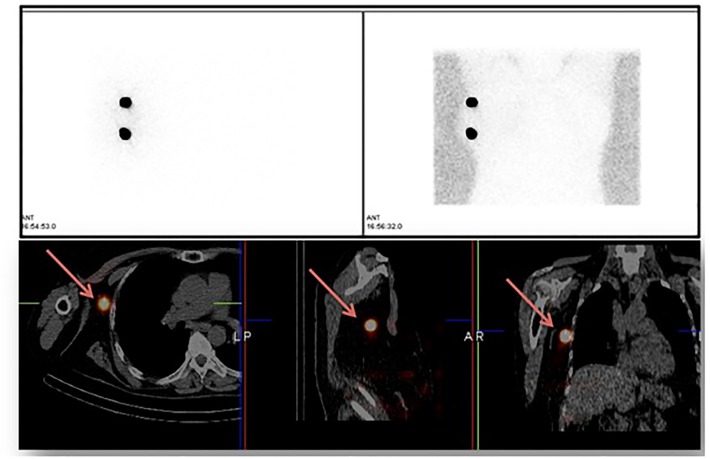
PS images in a female with right breast cancer showed no uptake in right axilla in addition to injection sites. SPECT/CT images localized tracer uptake in right level I axillary SLN
